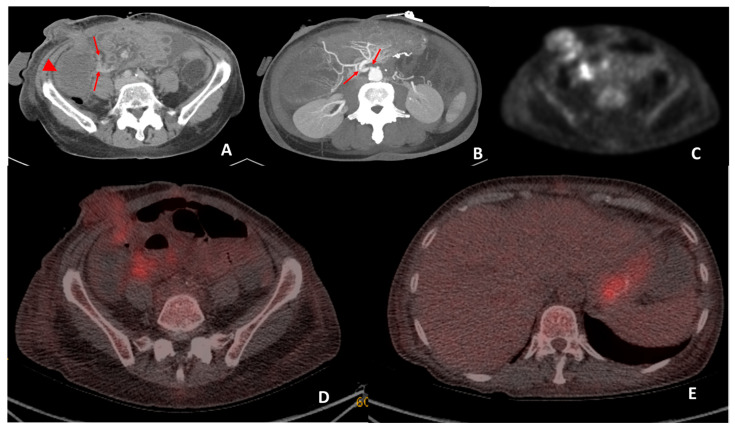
CT performed on day 19 post transplant for sepsis and query ischemia or rejection. Portal venous-phase CT imaging was performed on day 19 post transplant (A) demonstrating subtle mural oedema in the caecum (arrowhead) and sub centimeter mesenteric lymph nodes (arrows) surrounding the caecum. Maximum intensity projection (B) of the superior mesenteric origin on post-operative day 19 CT demonstrates a double kink (arrows) at this site. The patient subsequently underwent angiography and pressure measurements, which demonstrated a 27 mmHg pressure gradient between the aorta and SMA. Angioplasty was performed and reduced the pressure gradient to 21 mmHg. Attenuation-corrected ^18^F-FDG PET (C) demonstrated increased uptake in the mucosa of the small bowel surrounding the stoma and the mesenteric lymph nodes. Hybrid imaging ^18^F-FDG PET-CT demonstrated co-localisation of the increased uptake to the small bowel surrounding the stoma and also at the mesenteric lymph nodes (D). Biopsies performed on the small bowel surrounding the stoma were reported to be in keeping with mild rejection. The increased low-grade uptake in the stomach (E) was interpreted as physiological uptake.
